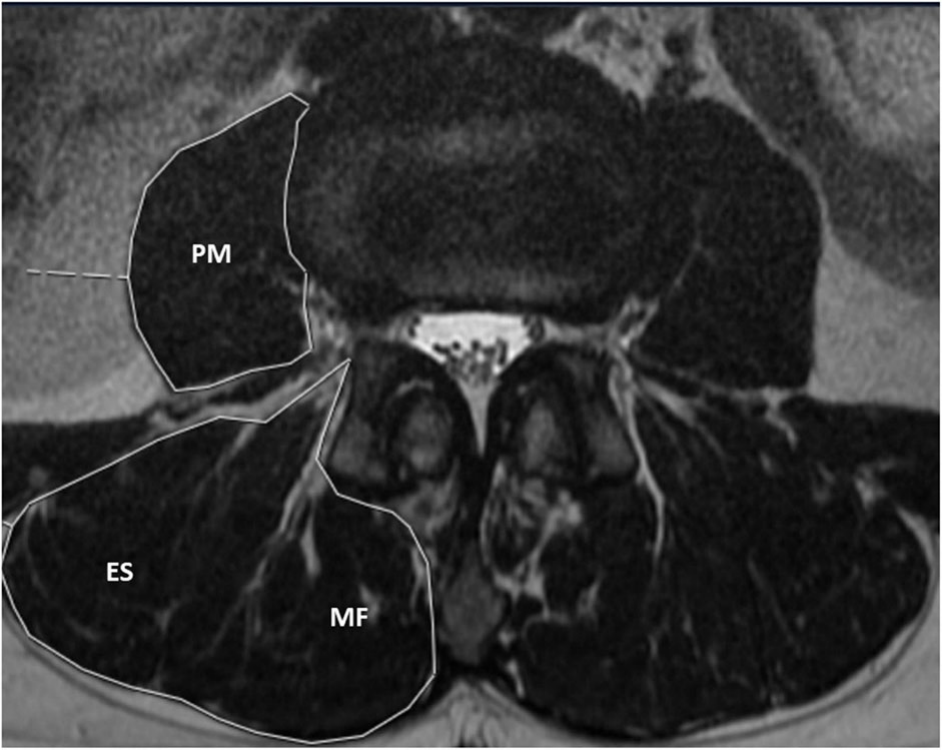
Axial T2-weighted magnetic resonance image obtained at the level of the upper endplate of L3. The muscle fat index (MFI) was calculated by dividing the mean signal intensity of the psoas major (PM) with the mean signal intensity of the erector spinae (ES) and the multifidus (MF) muscles
